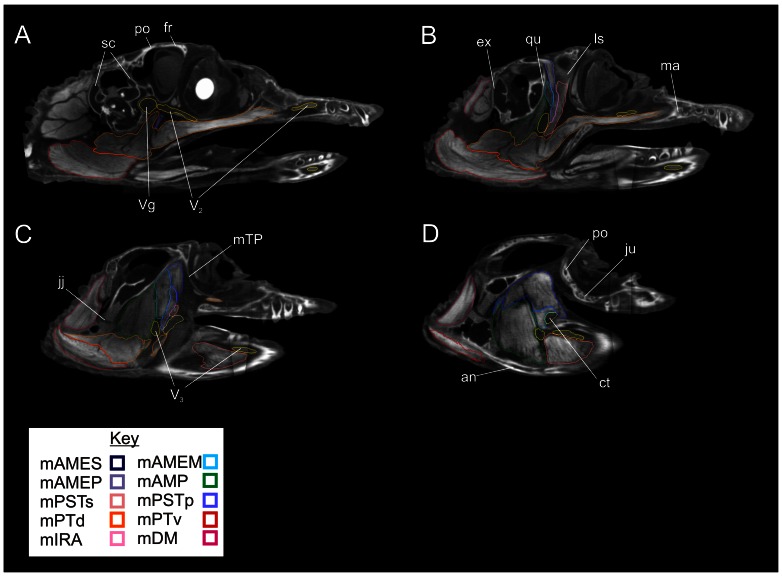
Lateral views of parasagittal sections through the segmented CT data showing labeled individual right jaw muscles. A, Slice 183/512; B, Slice 155/512; C, Slice 133/512; D, Slice 119/512. Abbreviations: an, angular; ct, cartilago transiliens; ex, exoccipital; ju, jugal; ls, laterosphenoid; ma, maxilla; mTP, m. tensor periorbitae; po, postorbital; qu, quadrate; sc, semicircular canals; V_2_, maxillary nerve; V_3_, mandibular nerve; Vg, trigeminal ganglion.
